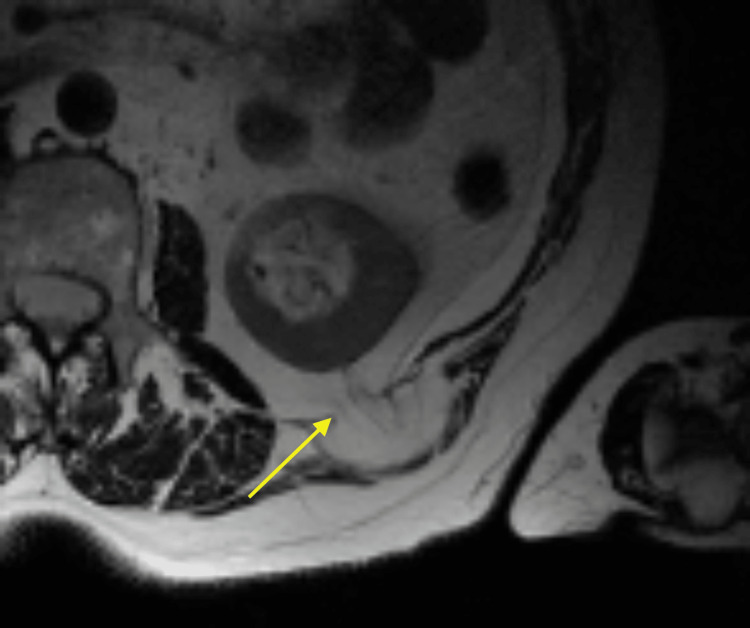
CT with an arrow showing a posterior perirenal hernia defect
